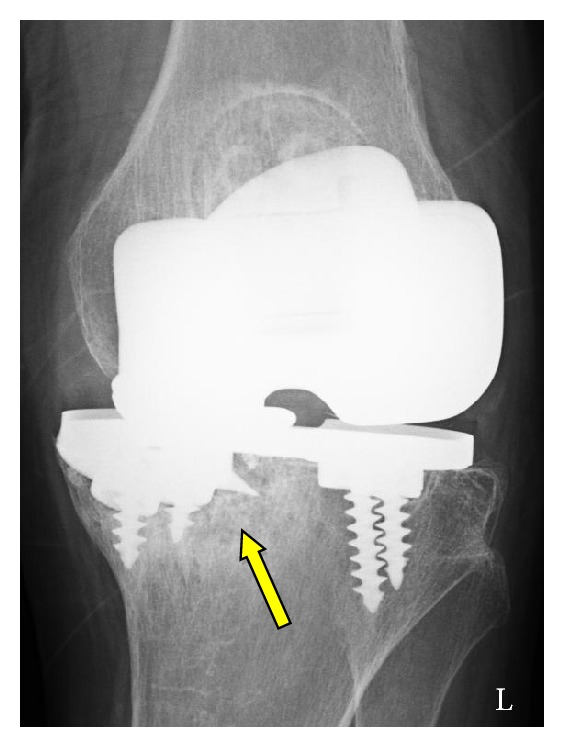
Follow-up plain radiograph obtained 16 years after the surgery shows disappearance of the medial joint space, subsidence of the broken baseplate, and osteolysis under the medial compartment (arrow).
